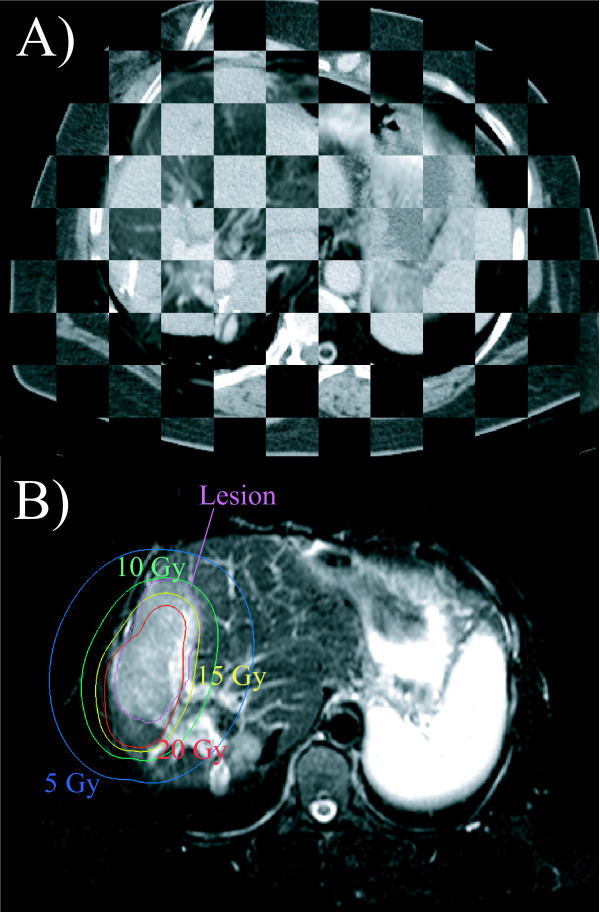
Image registration. A) T2-w image coregistered with the planning CT. Note that only the liver was coregistered and therefore good matching of the images was only achieved for the liver. B) T2-w image showing segmented lesion and isodoses at 12-week follow-up. A prononounced shift of the irradiation effect with respect to the planned dose distribution as shown in this example was typically not found.
